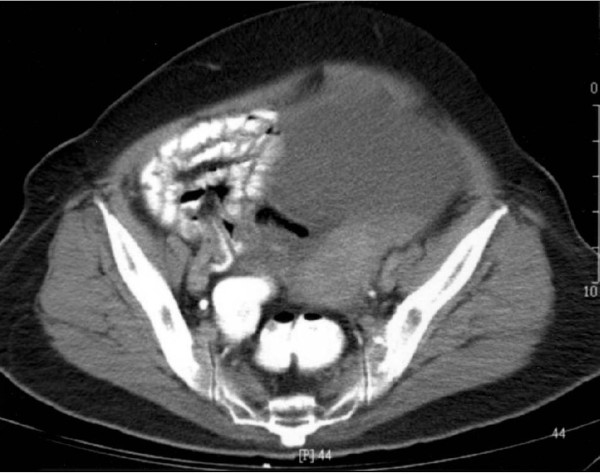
Abdominal computed tomography shows homogeneous cyst on the right side of the pelvis, which was larger than 35 mm in maximal diameter with a solid component.
